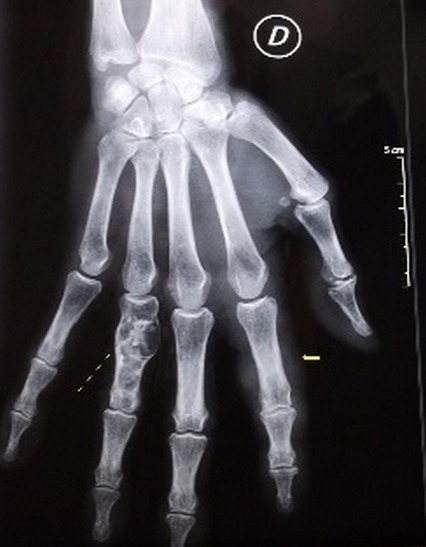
Radiographie standard de la main droite: épaississement des partie molles du 2ème rayon droit+ une lésion diaphysaire de la 4ème IPP droite, lytique et condensante, cernée d'un fin liseré d'ostéosclérose avec une corticale soufflée compatible avec un chondrome
